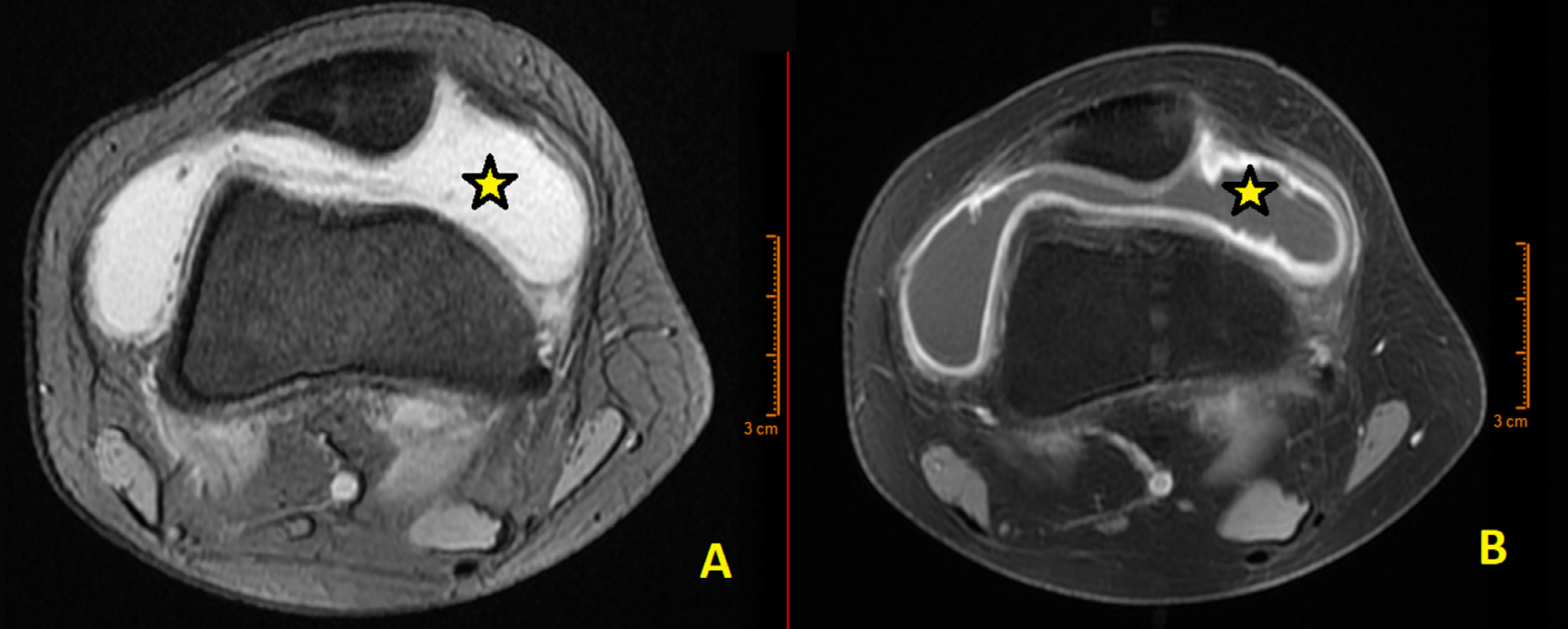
Axial MRI of the left knee demonstrating diffuse intra-articular Pigmented Villonodular Synovitis (PVNS). (A) T2-weighted multi-echo gradient image showing a multinodular lesion with low signal intensity due to hemosiderin deposits. The image reveals extensive synovial thickening and nodular formations, with the hemosiderin deposits contributing to the observed signal drop-out effect typical of PVNS. (B) T1-weighted, fat-saturated postcontrast sequence displaying enhanced synovial proliferation with marked contrast uptake, highlighting the extent of synovial thickening and nodularity. The stars indicate the villous and nodular lesions, which are prominent due to the contrast enhancement and low signal intensity areas from hemosiderin deposits.
